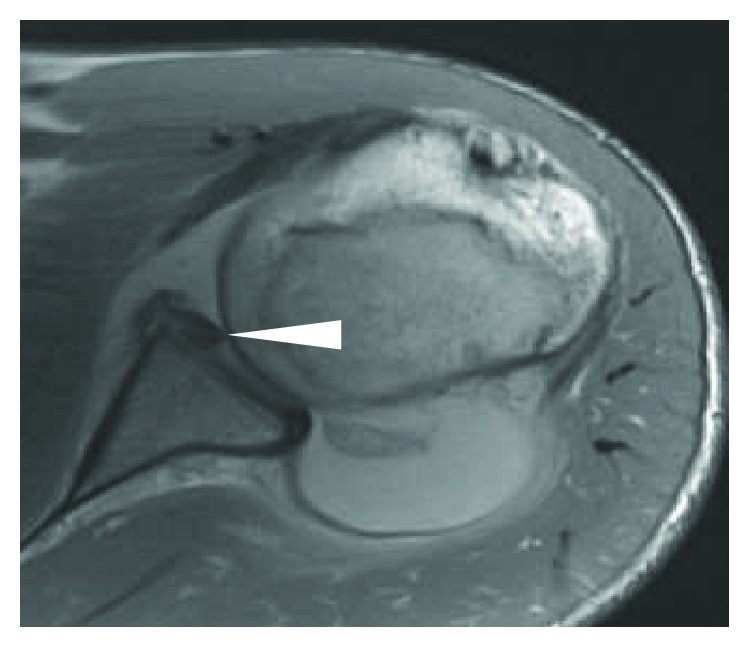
Axial T1 weighted MRI shoulder slice of the left shoulder with an arrow pointing to the everted fragment.
